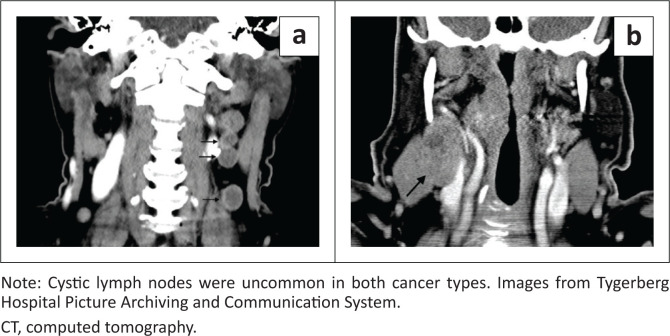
Coronal contrast enhanced neck CT images showing morphologically: (a) cystic and (b) necrotic nodes.
